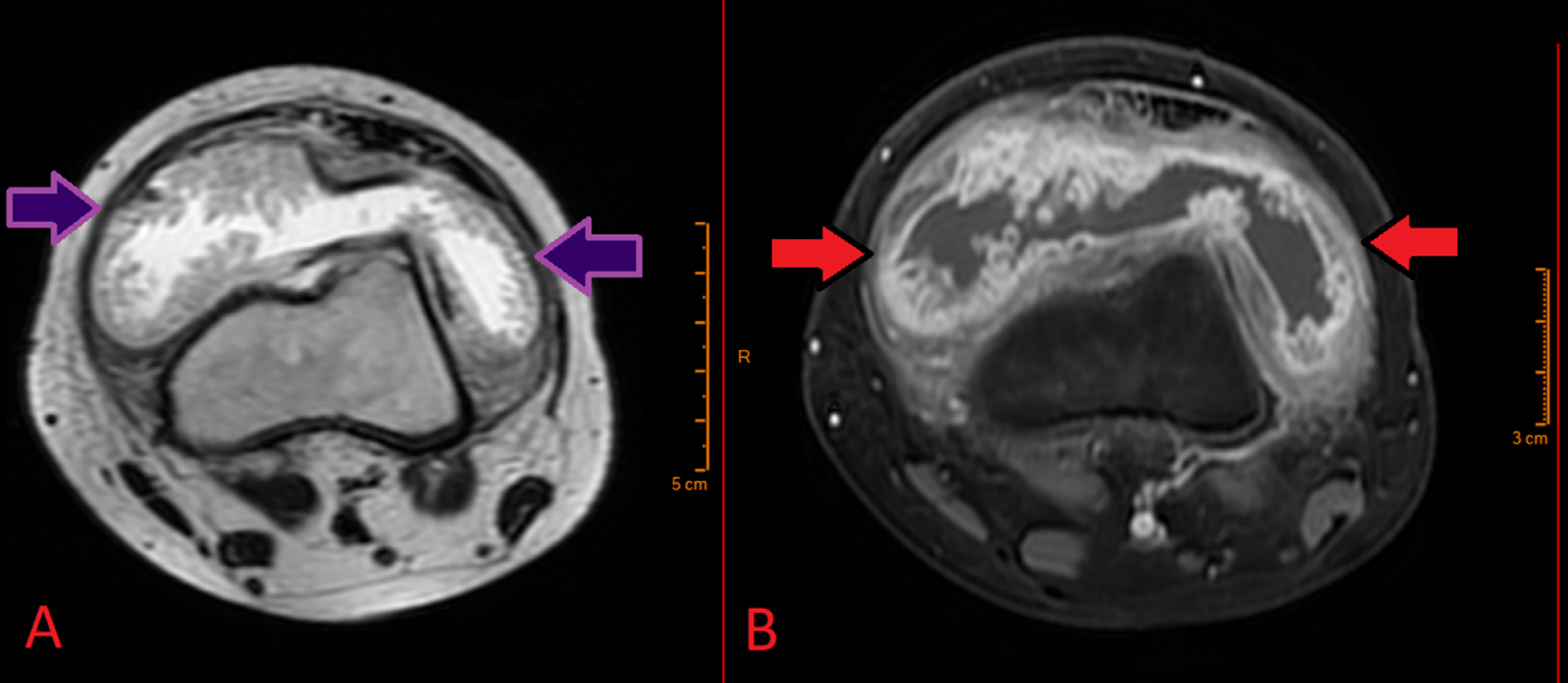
Axial MRI of the right knee demonstrating diffuse intra-articular pigmented villonodular synovitis (PVNS). (A) T2-weighted image showing a multinodular lesion with low signal intensity due to the presence of hemosiderin deposits. The lesion is characterized by diffuse synovial thickening and irregular nodular areas. (B) T1-weighted, fat-saturated postcontrast sequence revealing enhanced synovial proliferation with marked contrast uptake indicating active disease. The arrows highlight the extent of synovial thickening and nodularity, with hemosiderin deposits visible as areas of low signal intensity.
